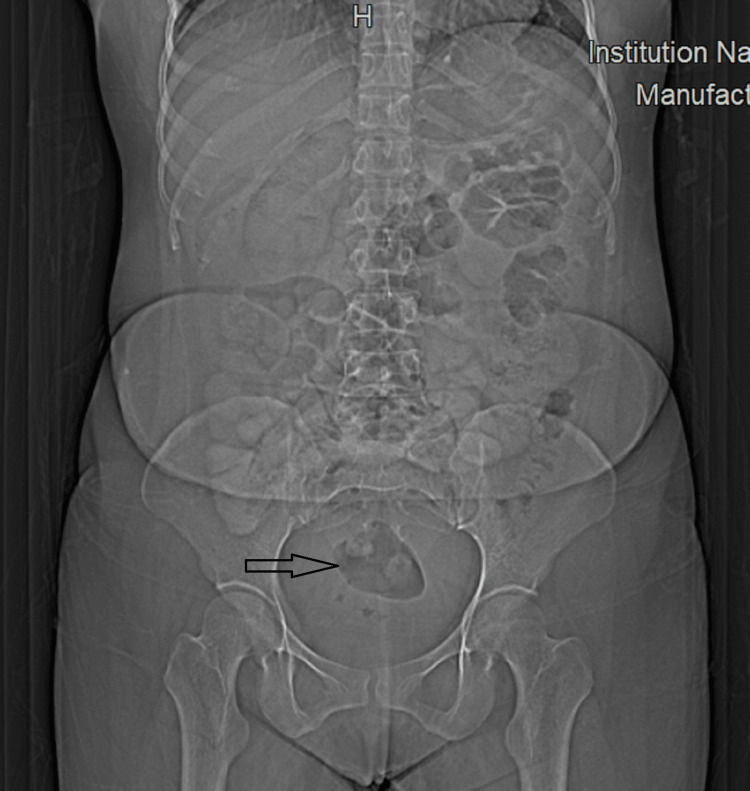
Normal abdominal X-ray pattern of the patient with gas shadow reaching till the rectum marked by asterisk
